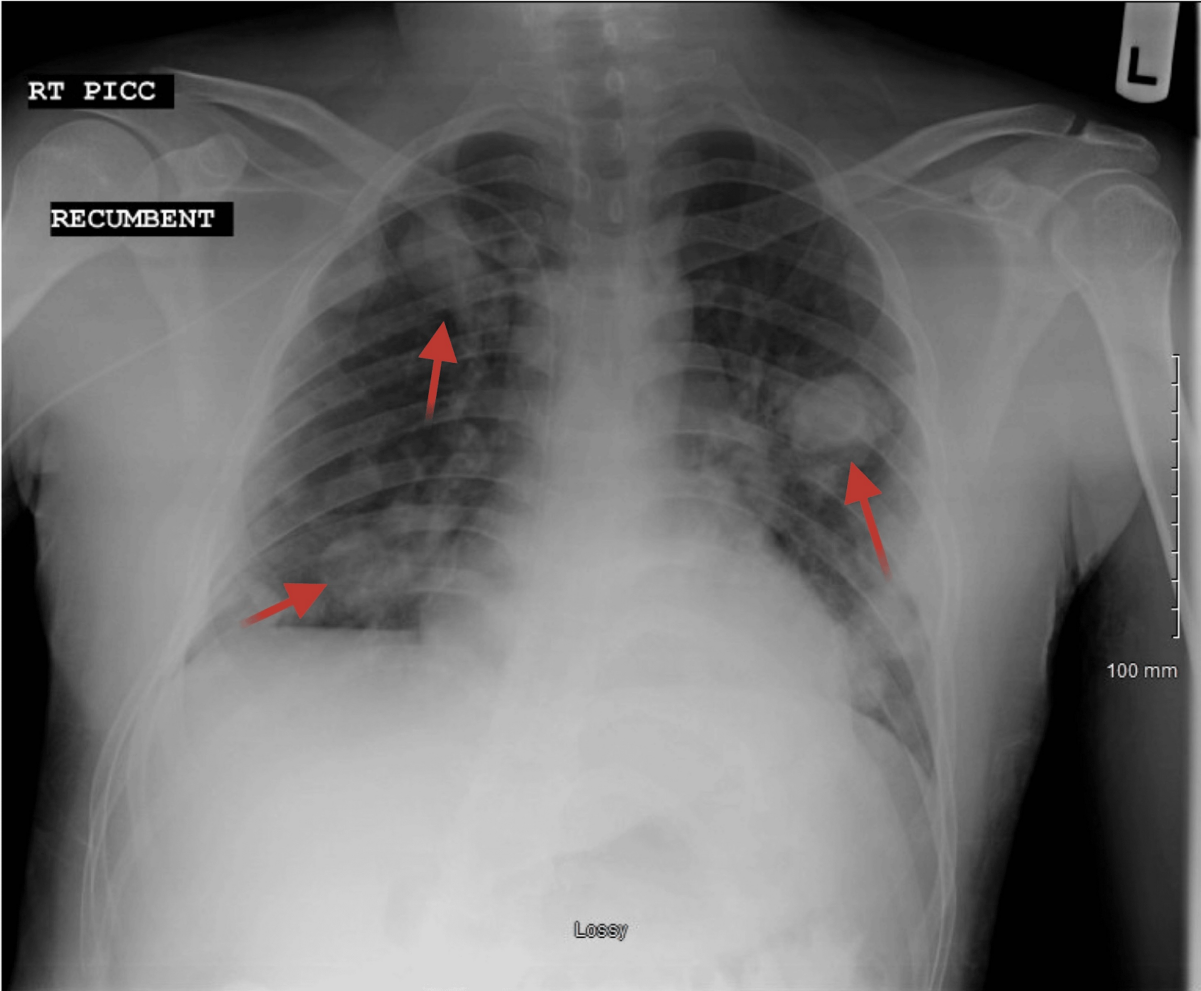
X-ray of the chest anteroposterior portable - multiple masses in both lungs
